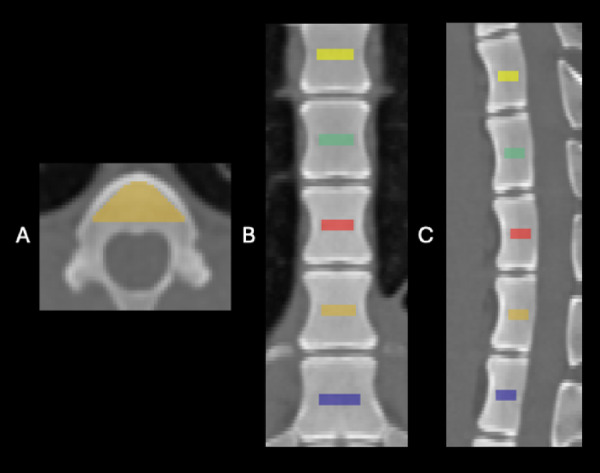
Placement of the regions of interest (ROI) in the vertebral bodies of a Göttingen minipig in a non-contrast CT examination with a radiation dose of 10.01 mGy in A axial, B coronal and C sagittal view. Th9 is annotated in brown. mGy = milligray.
